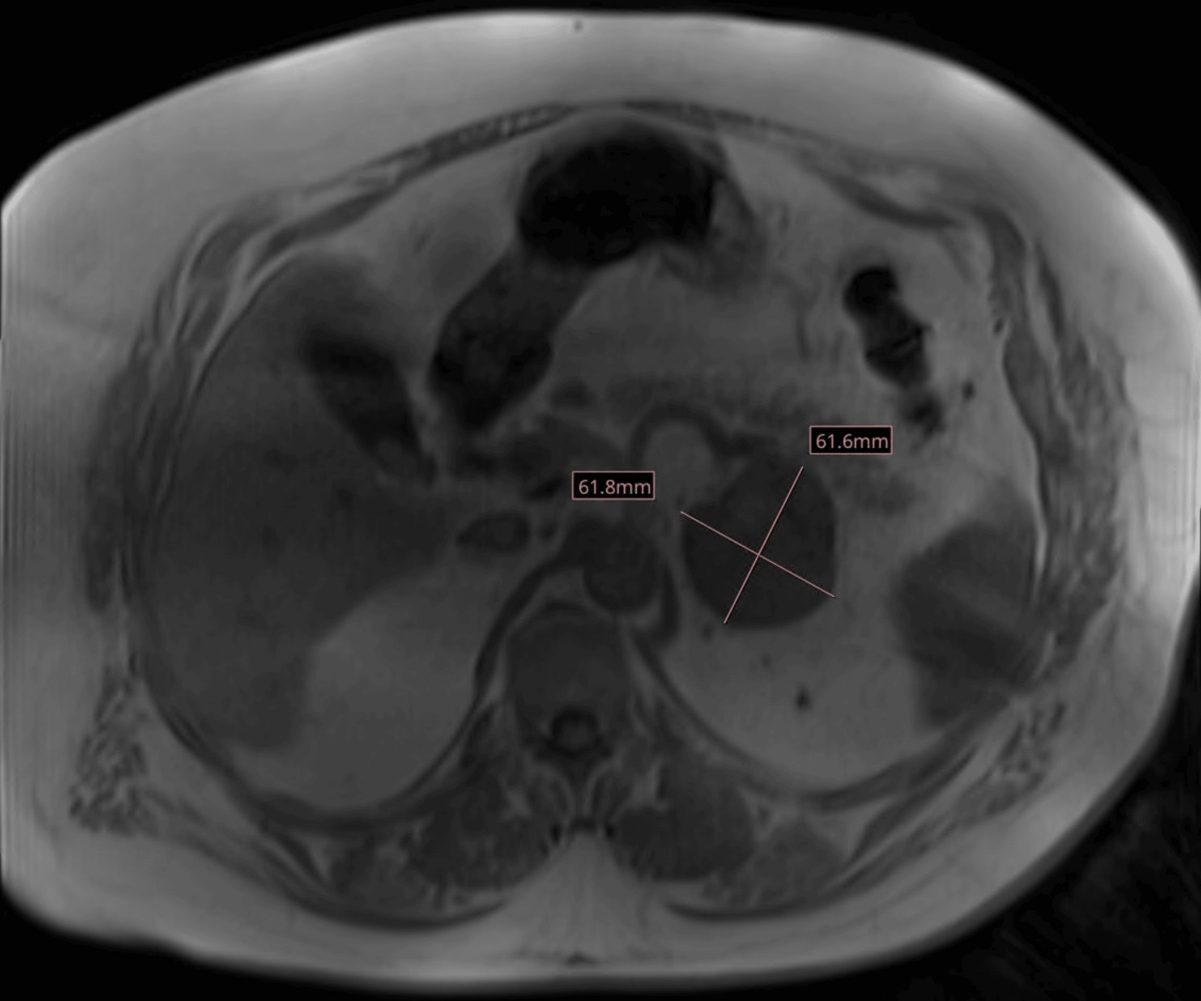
Abdominal MRI showing interval enlargement of the mass to 6.2 x 6.2 cm.
